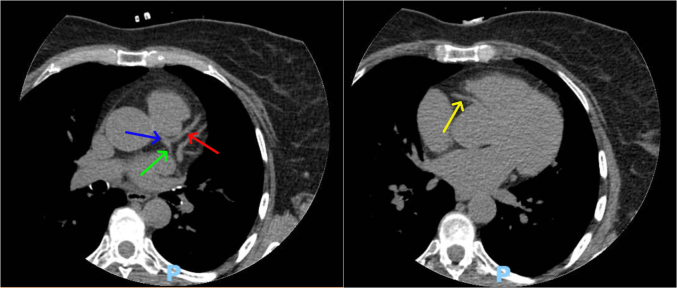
Calcium Score on Computed Tomography Showing Absence of Coronary Calcifications in the LMCA (blue arrow), LAD (red arrow), LCx (green arrow), and RCA (yellow arrow) LAD = left anterior descending artery; LCx = left circumflex artery; LMCA = left main coronary artery; RCA = right coronary artery.
